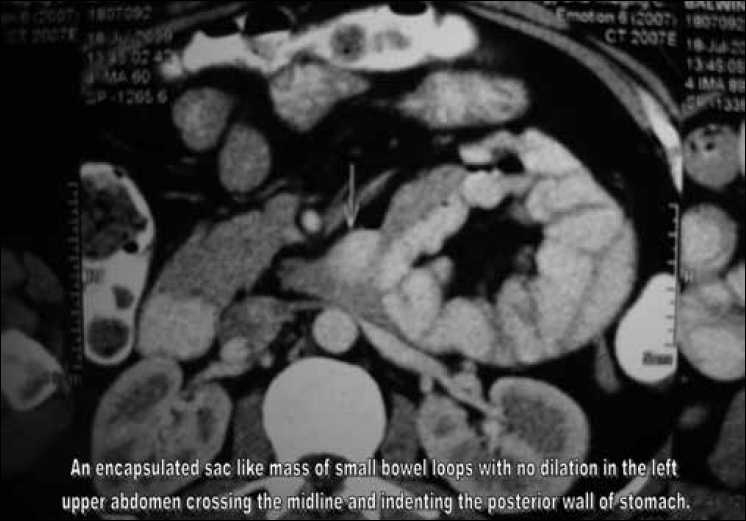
CT scan showing an encapsulated sac
